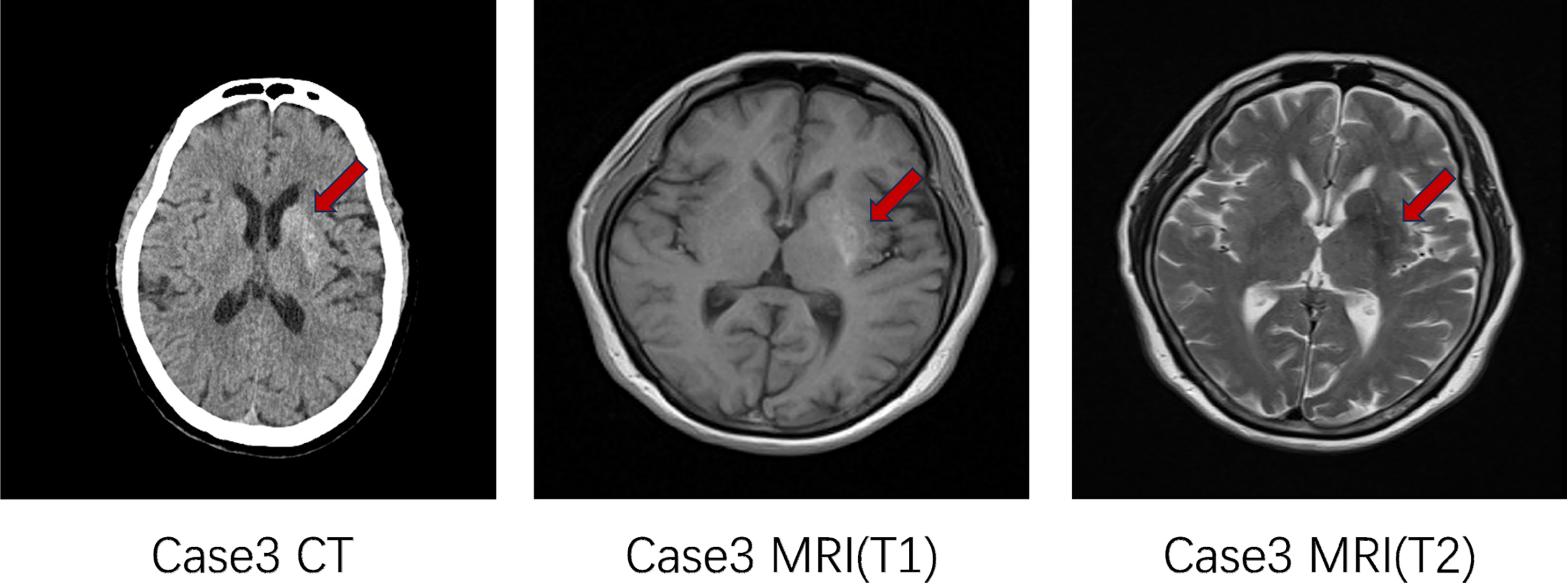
Imaging manifestation of case 3. CT, computed tomography; MRI, magnetic resonance imaging.
